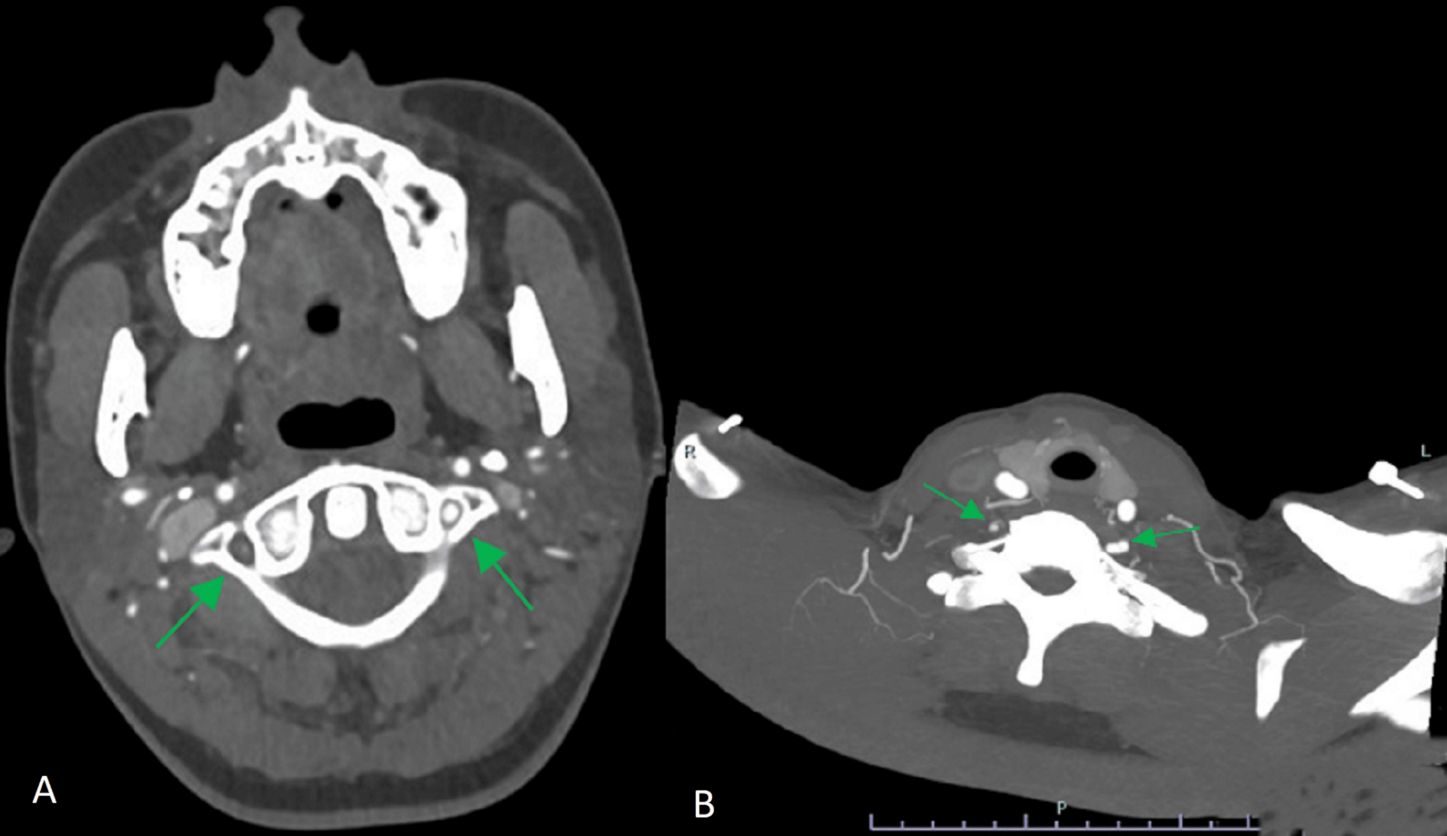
(A) and (B) CTA images showing loss of contrast flow within the right vertebral artery, with evidence of an intimal flap suggesting VAD. The left vertebral artery demonstrates normal opacification and caliber. CTA, computed tomography angiography; VAD, vertebral artery dissection.
